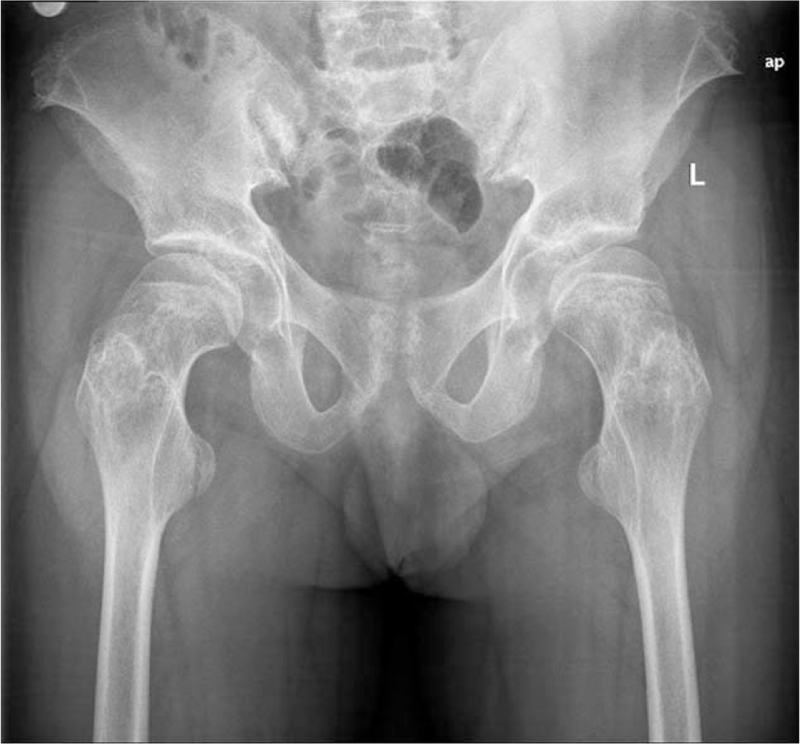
Anteroposterior radiograph of the hips demonstrates bilateral unfused femoral proximal epiphyses and ossification centers of the lesser trochanters.
